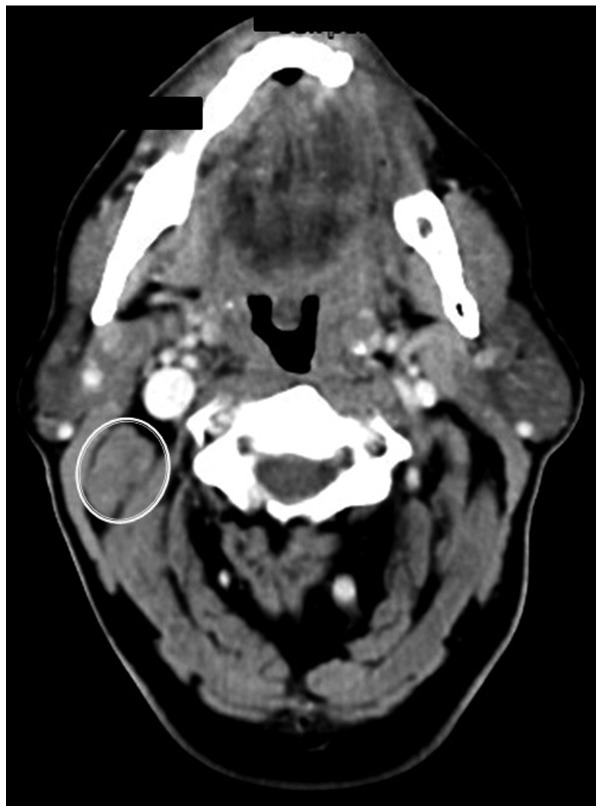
Computed tomography (CT) scan showing a nodular mass in the right cervical space (circle).
